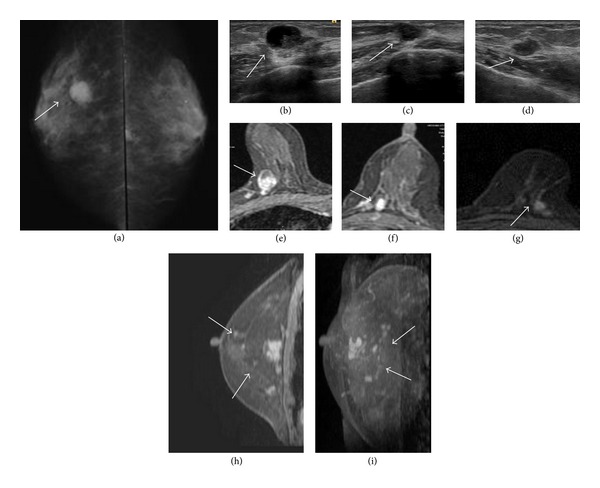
Mammogram showing a round, high-density mass in the right breast ((a), arrow). Ultrasound view showing tumor 1 in the right breast (b) and tumors 2 and 3 in the left breast ((c), (d), resp.; arrows). Magnetic resonance imaging (MRI) scans showing all of these tumors ((e), (f), and (g); arrows) with diffuse nodules surrounding the tumors in the bilateral breast ((h): left; (i): right; arrows).
